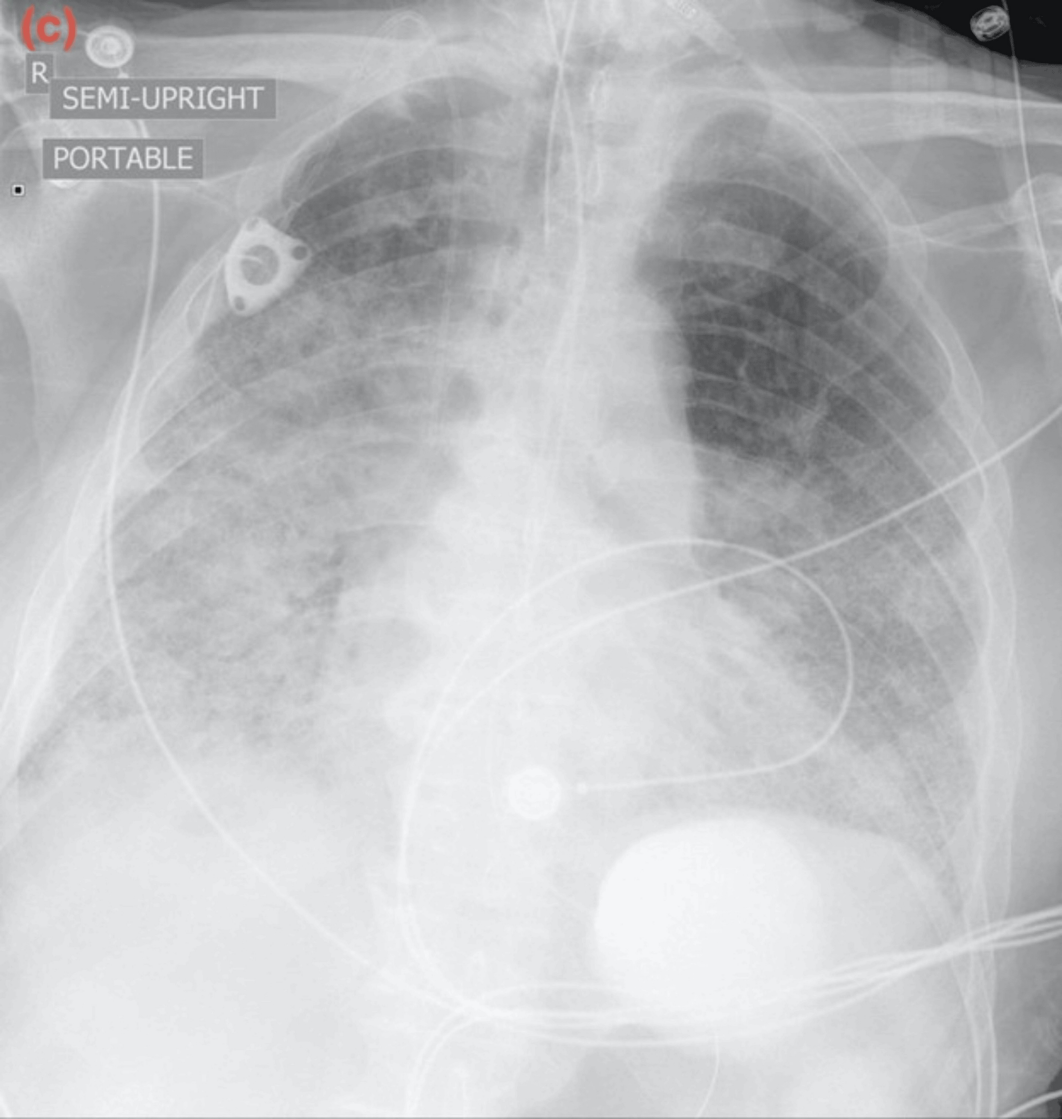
Progression of the disease on chest X-ray: (c) Chest X-ray after two weeks of hospital admission showing worsening of the bilateral infiltrates.
